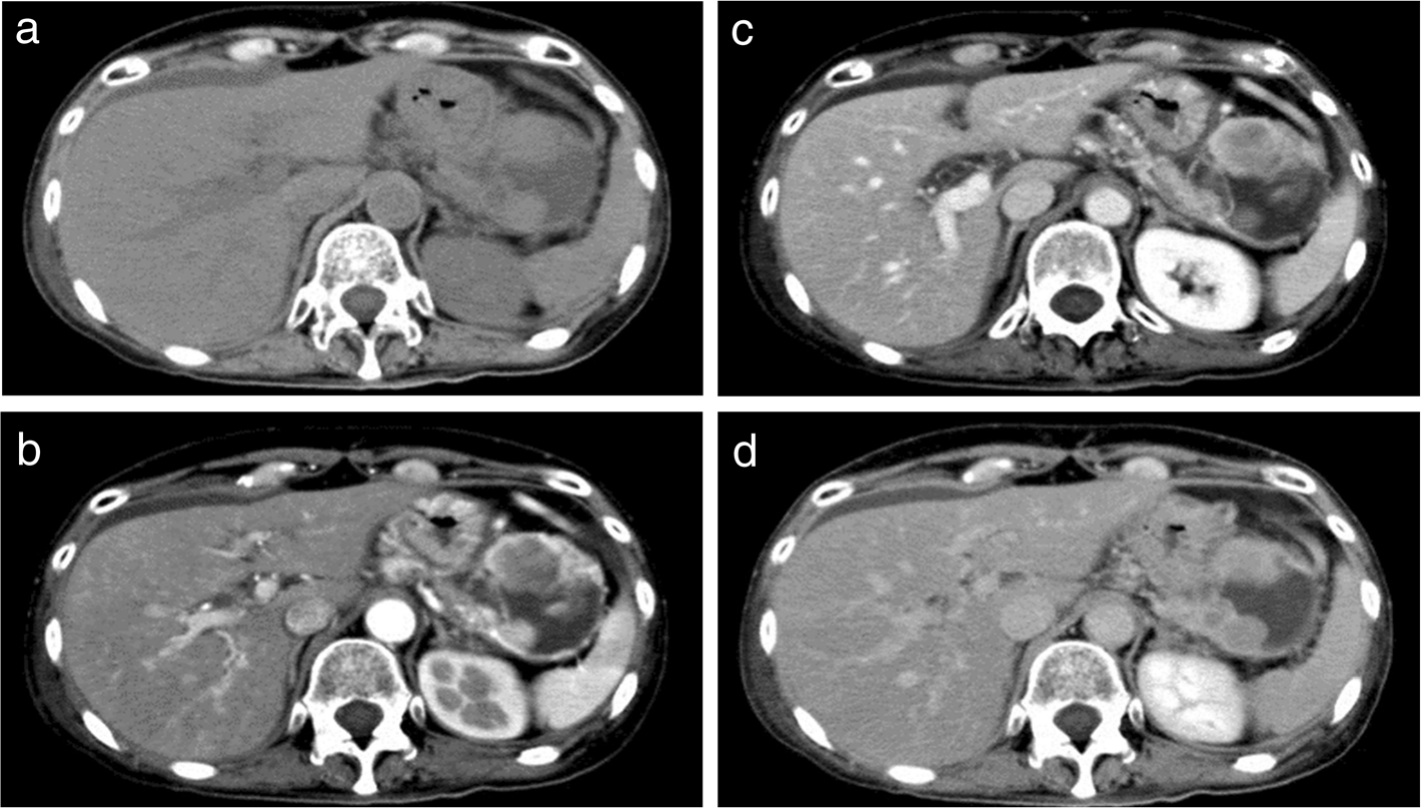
Dynamic contrast-enhanced computed tomography (CT) findings. a Plain CT scan demonstrated a 5.5-cm cystic mass with solid components inside in the pancreas tail. b Dynamic CT arterial phase scan demonstrated enhancement in the tumor rim and in the edge of the solid components with non-enhanced interior. c Solid components were gradually enhanced from the outside in the portal phase. d The tumor rim and the solid components had isodensity to the surrounding pancreatic parenchyma in the delayed phase
